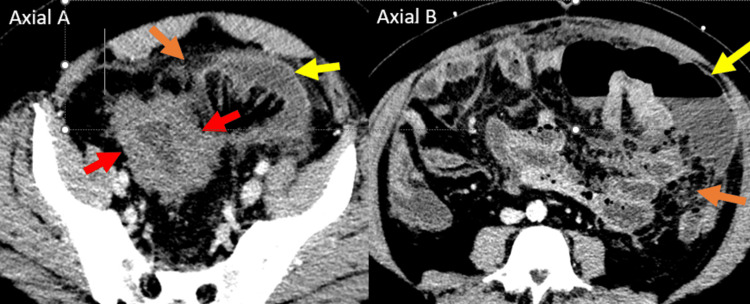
CT abdomen and pelvis with IV contrast: Axial image through the level of the sigmoid colon (A) shows wall thickening (red arrows) with surrounding fat stranding (orange arrow) and small crescentic extraluminal fluid collection (yellow arrows). A superior axial image (B) shows a larger free intraperitoneal fluid and air collection (yellow arrows) consistent with perforation.
